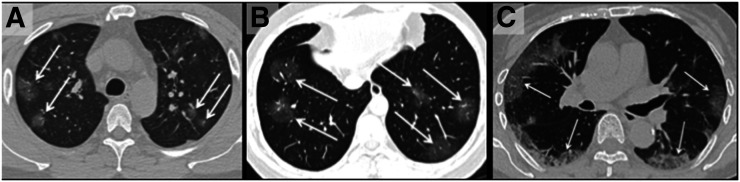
(A) Unenhanced axial CT image from 36‐year‐old man shows bilateral ground‐glass opacities in upper lobes with rounded morphology (arrows). (B) Axial CT image from 44‐year‐old man shows larger ground-glass opacities bilaterally in lower lobes with rounded morphology (arrows). (C) Axial CT image from 65‐year‐old woman shows bilateral ground‐glass and consolidative opacities with striking peripheral distribution. (Reprinted with permission of (32).)
